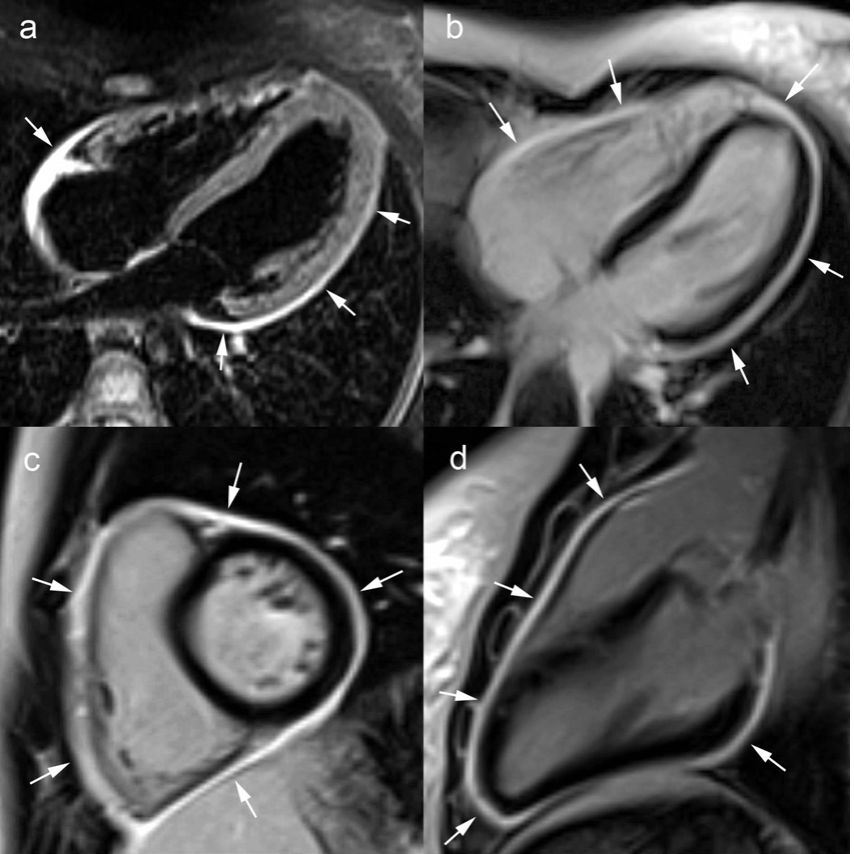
Acute viral inflammatory pericarditis in 15-year-old girl. T2-weighted short-tau inversion recovery spin-echo CMR (a), LGE CMR in horizontal long-axis (b), short-axis (c) and vertical long-axis plane (d). Diffuse hyperintense appearance of the pericardium on T2-weighted short-tau inversion recovery spin-echo CMR (arrows)(a). Strong, homogeneous enhancement of the entire pericardium following gadolinium administration (arrows)(b, c, d).
